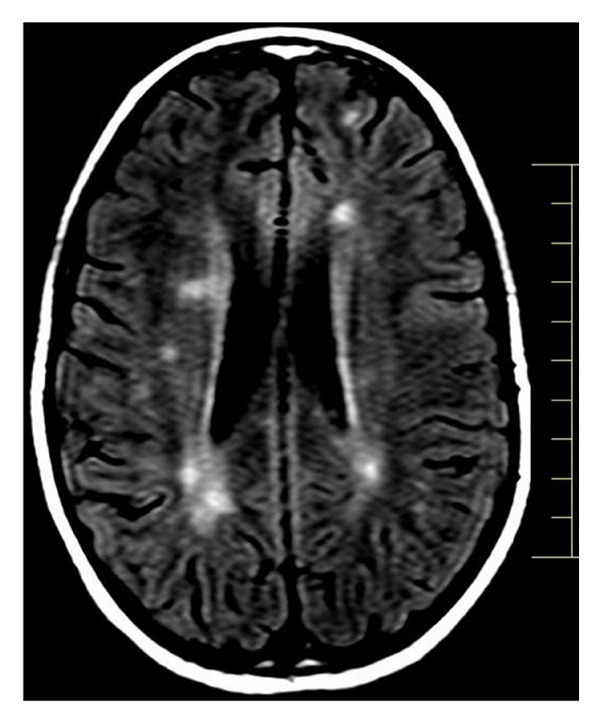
Brain MRI showing multiple periventricular lesions.
